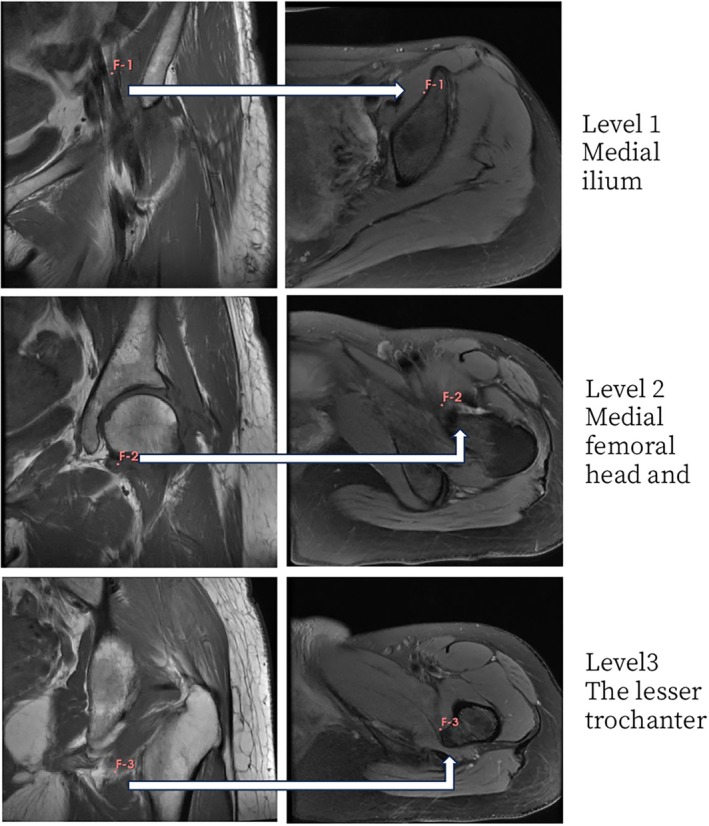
Standardized axial T1‐weighted MRI levels for iliopsoas assessment. Level 1: Medial ilium. Level 2: Medial femoral head and neck. Level 3: Lesser trochanter.
